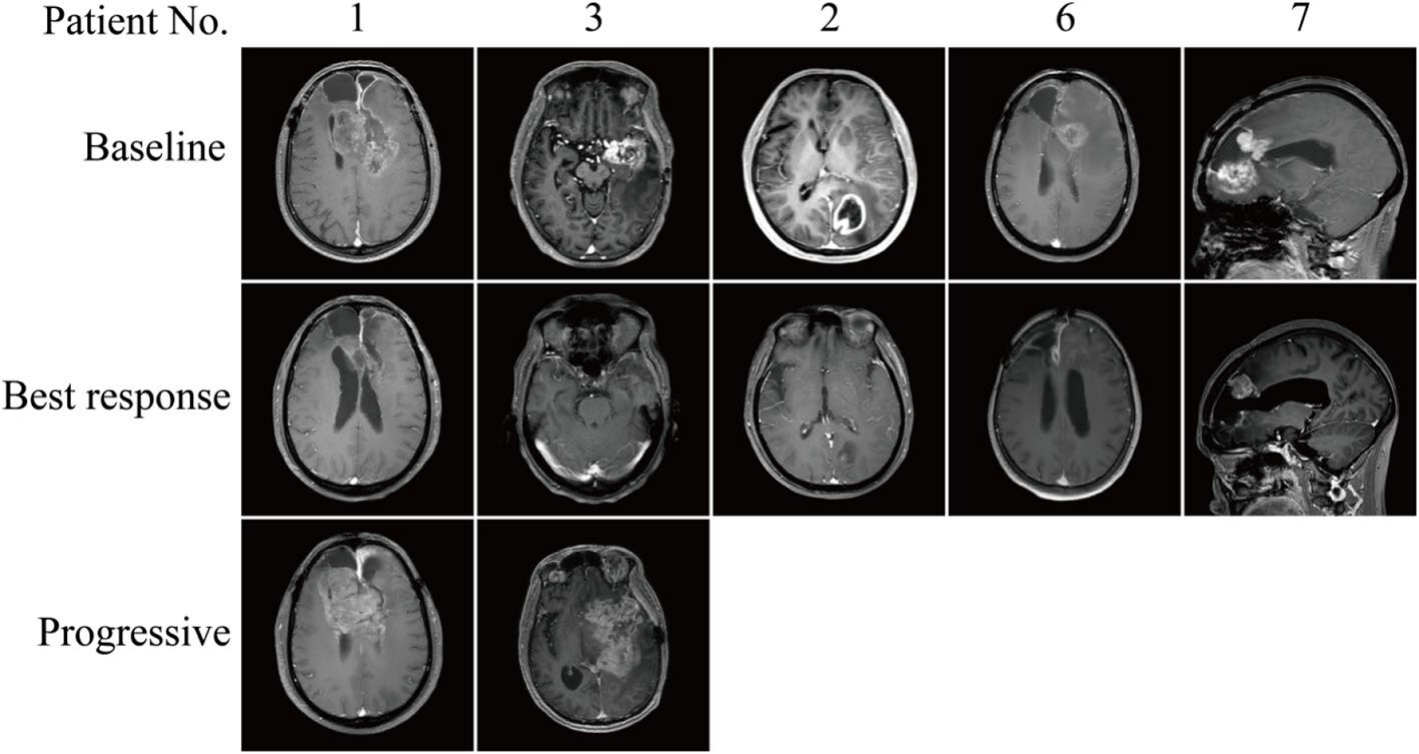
MR images(all T1-weighted images)at baseline, at the time of optimal efficacy and at the time of progression in five patients who achieved partial remission (MR at the time of relapse was not recorded in three of these patients due to loss to follow-up)
